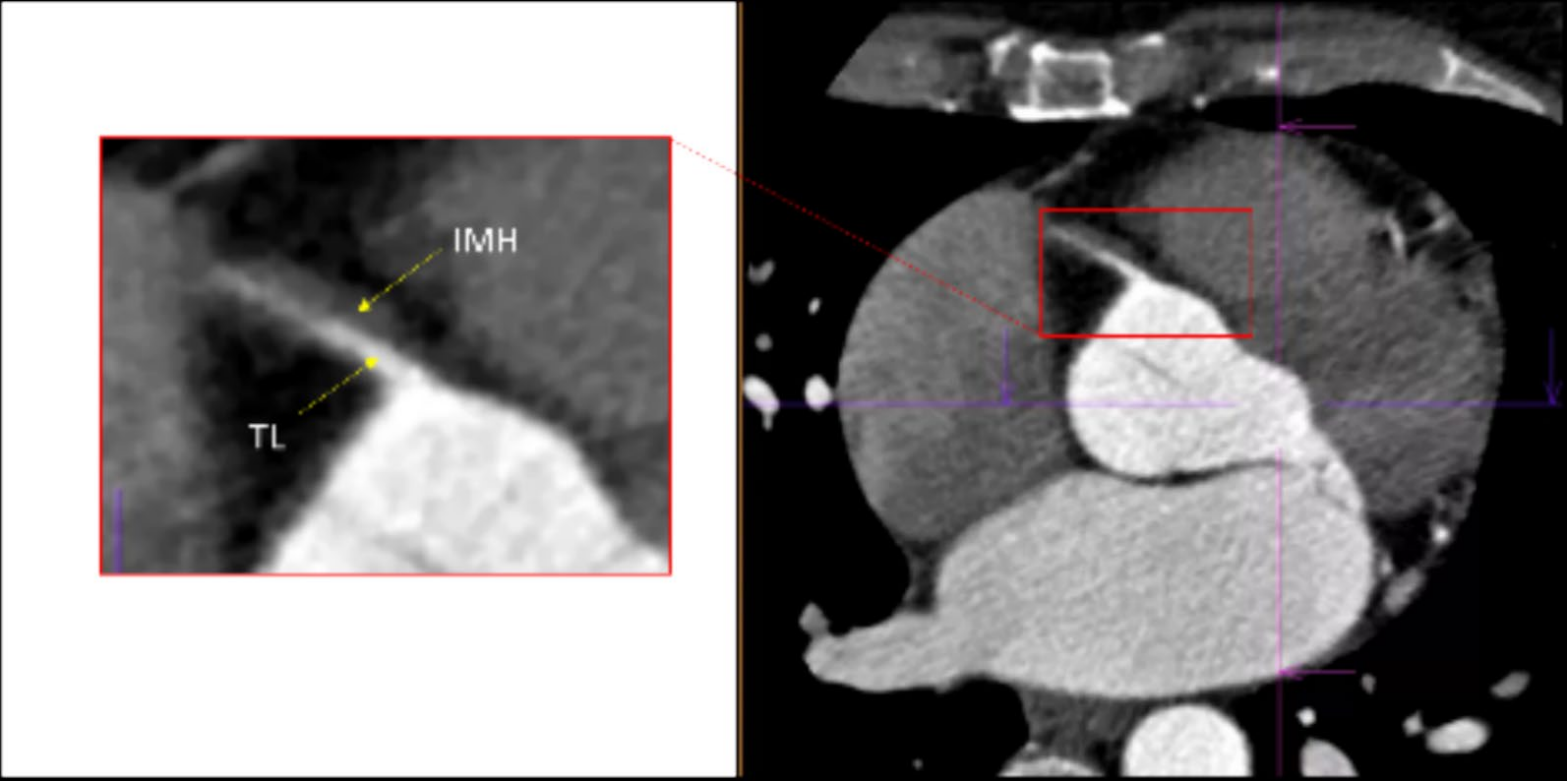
Cardiac computed tomography angiography. CCTA clearly depicts the TL and the IMH ruling out potential coronary occlusion and, above all, confirming RCA ostium sparing, assuring us about progression of the disease to the ascending aortas
